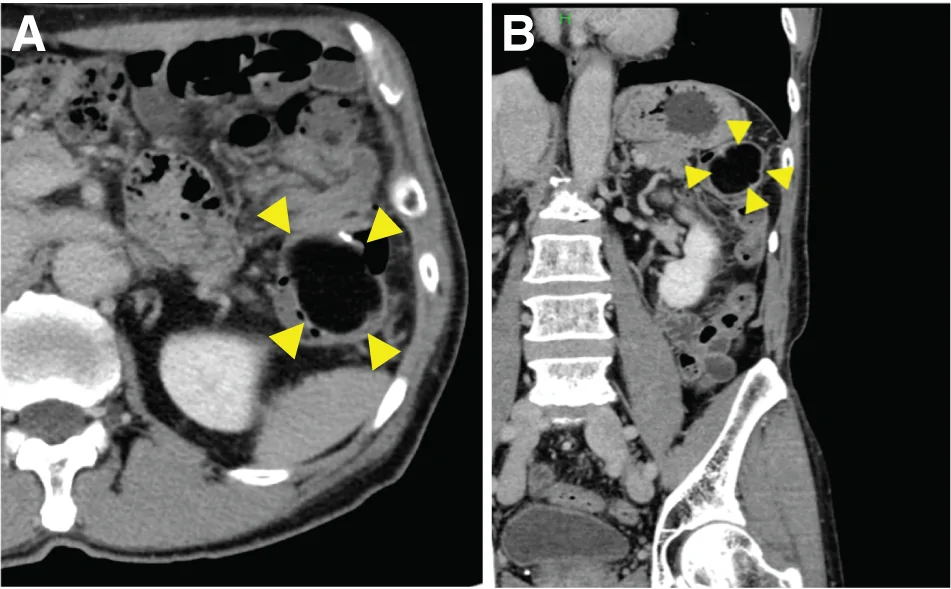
Contrast-enhanced CT findings. A 35 × 30-mm lipoma was identified on contrast-enhanced CT (yellow arrowhead). (A) Axial view; (B) coronal view.
